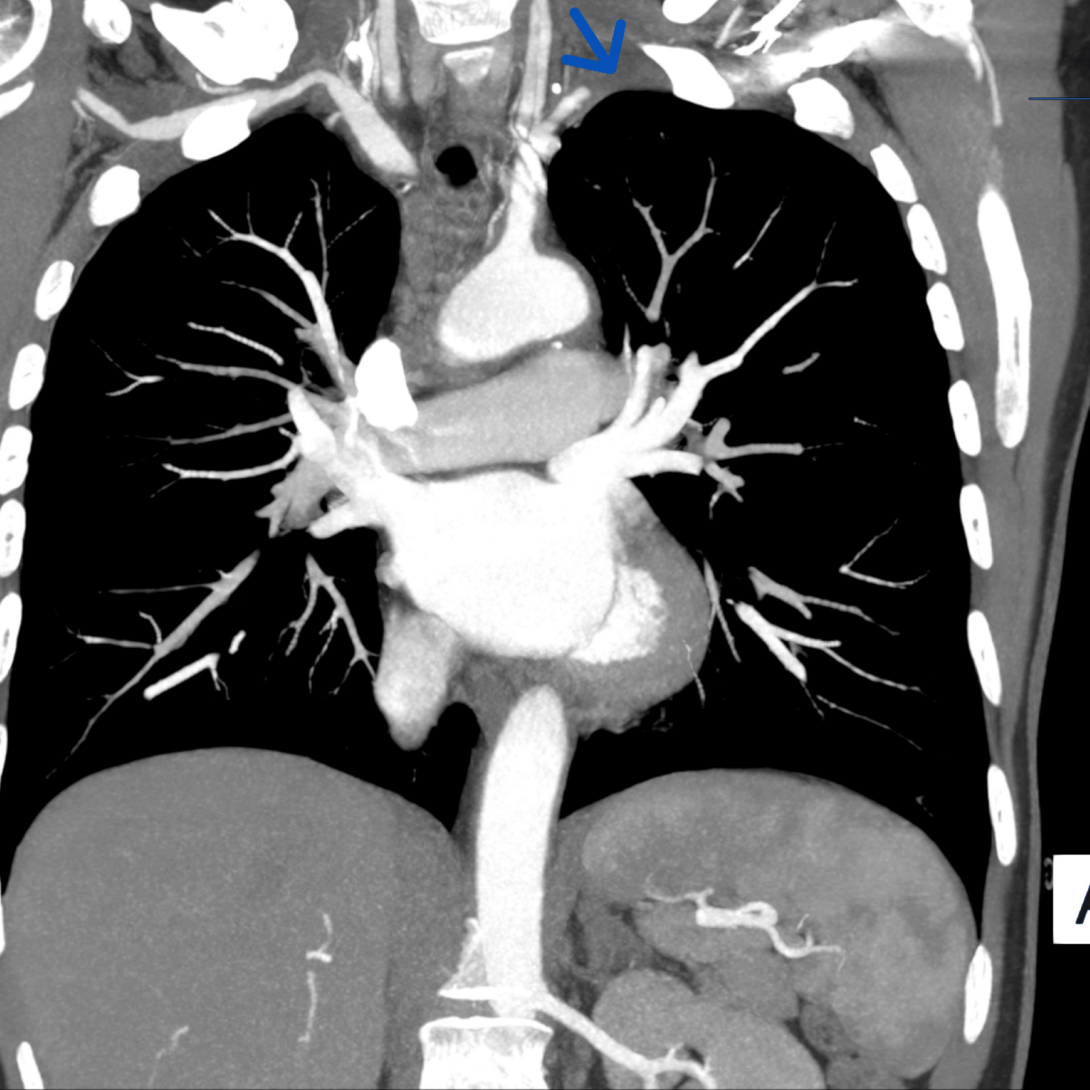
Contrast-enhanced CT TAP showing complete occlusion of the left subclavian artery (blue arrow) approximately 5 cm distal to its origin from the aortic arch. The absence of distal contrast opacification confirms vascular occlusion, a hallmark feature of LVV in this patient, and explains the clinical symptom of left arm claudication. CT TAP: CT of the thorax, abdomen, and pelvis
